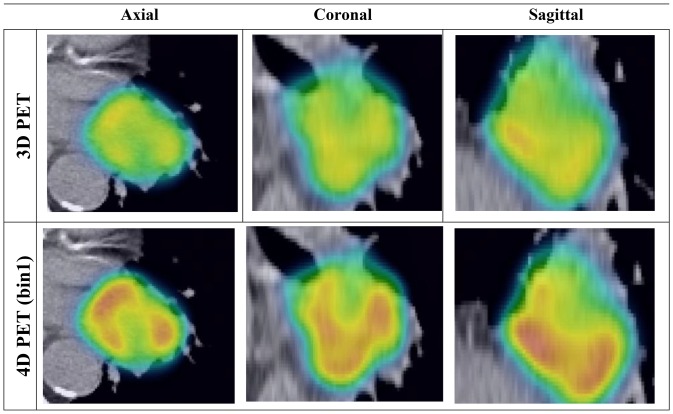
3D (top row) and 4D (bottom row) PET images overlaid onto the 3D CT. All images are displayed in the same intensity window with SUV between 1 and 15.
